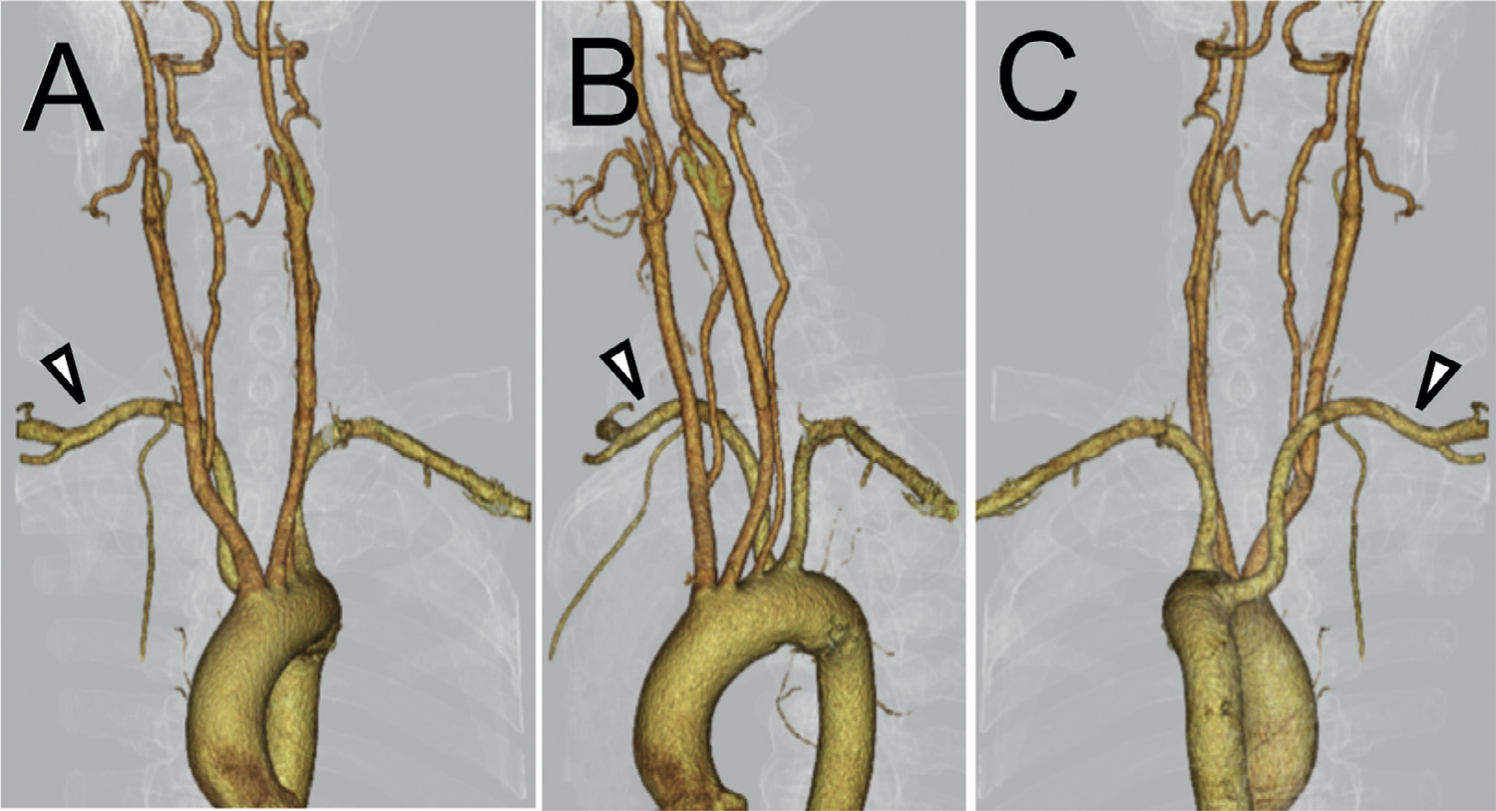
3D-CTA of the chest in anteroposterior (A), left lateral (B), and posteroanterior (C) projection confirms the presence of an aberrant RSCA (arrowhead). The aberrant RSCA arises from the descending aorta in the superoposterior direction.
